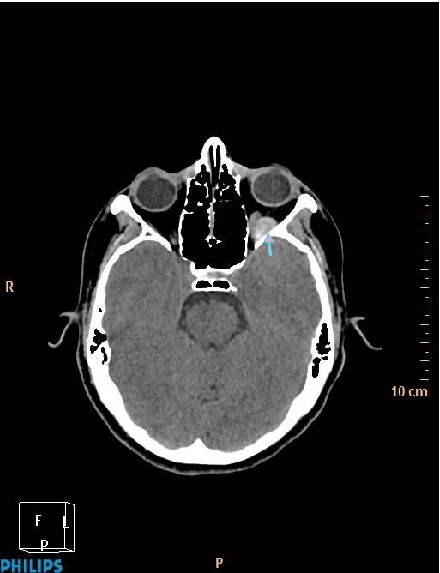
Axial postoperative CT image showing a small reduction in the size of haematoma (arrow).
